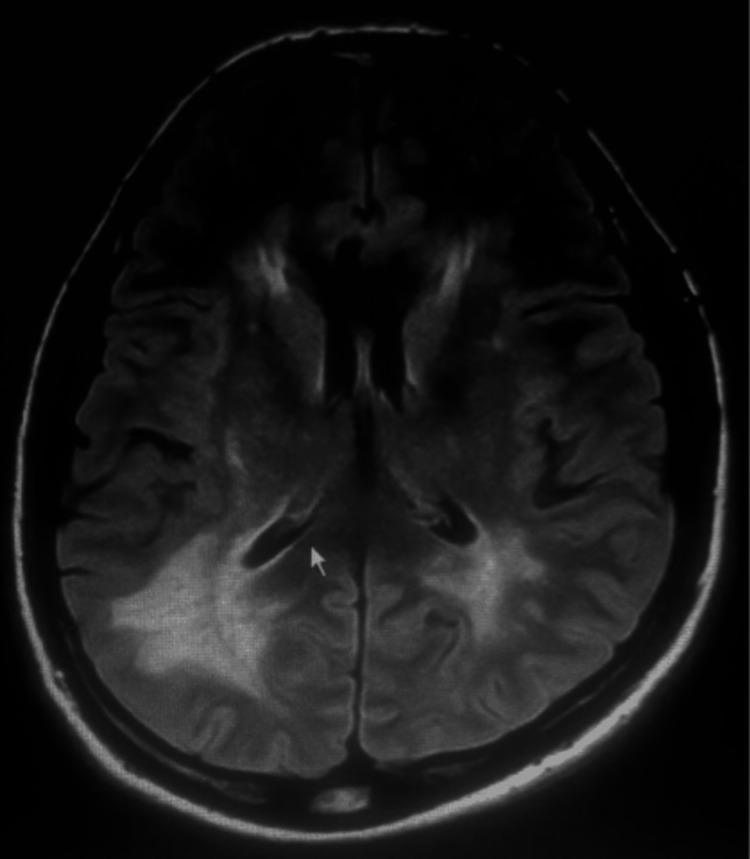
MRI indicating idiopathic intracranial hypertension
